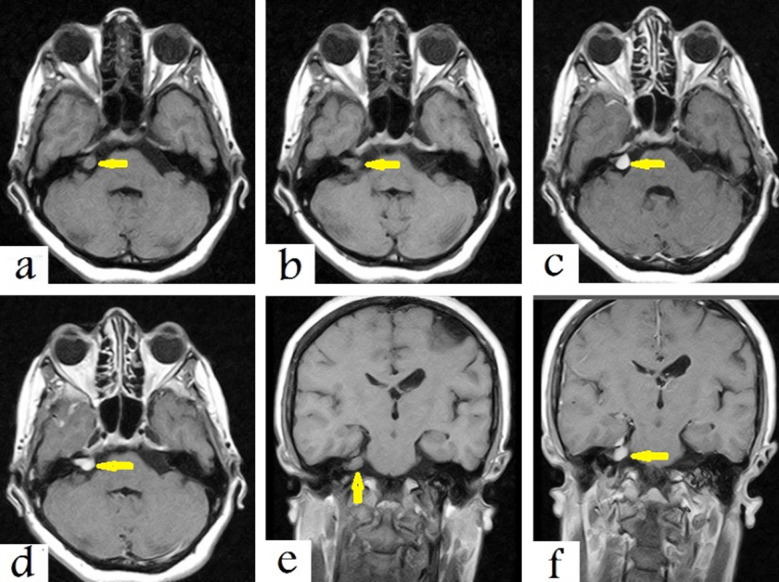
Selected images of a brain MRI, a, b) Axial T1-weighted images show a well-defined Isointense mass in the right cerebellopontine angle (arrows) with meatal extension along the right vestibular nerve, c, d) Contrast enhanced T1-weighted images show avid mass enhancement after contrast administration (arrows), e, f) Coronal T1-weighted images pre- and post-contrast show avid mass enhancement after contrast, which is typical feature of right vestibular schwannoma (arrows)..
